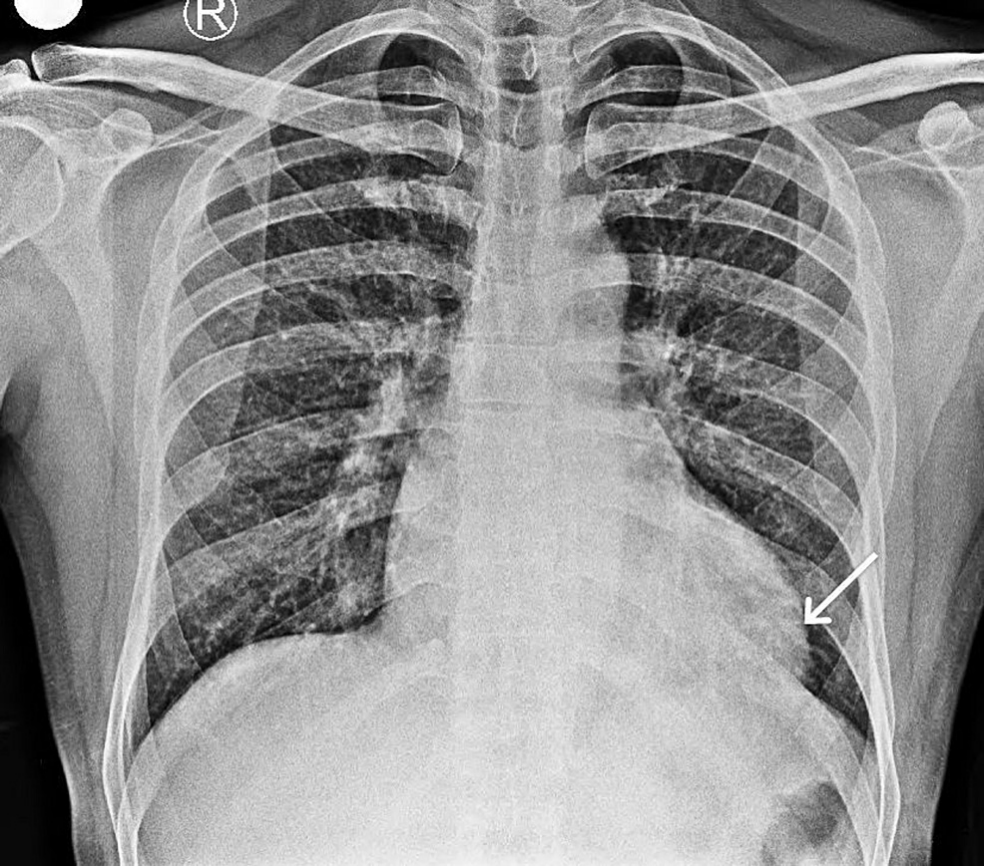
Chest roentgenogram Arrow pointing left ventricular enlargement.
